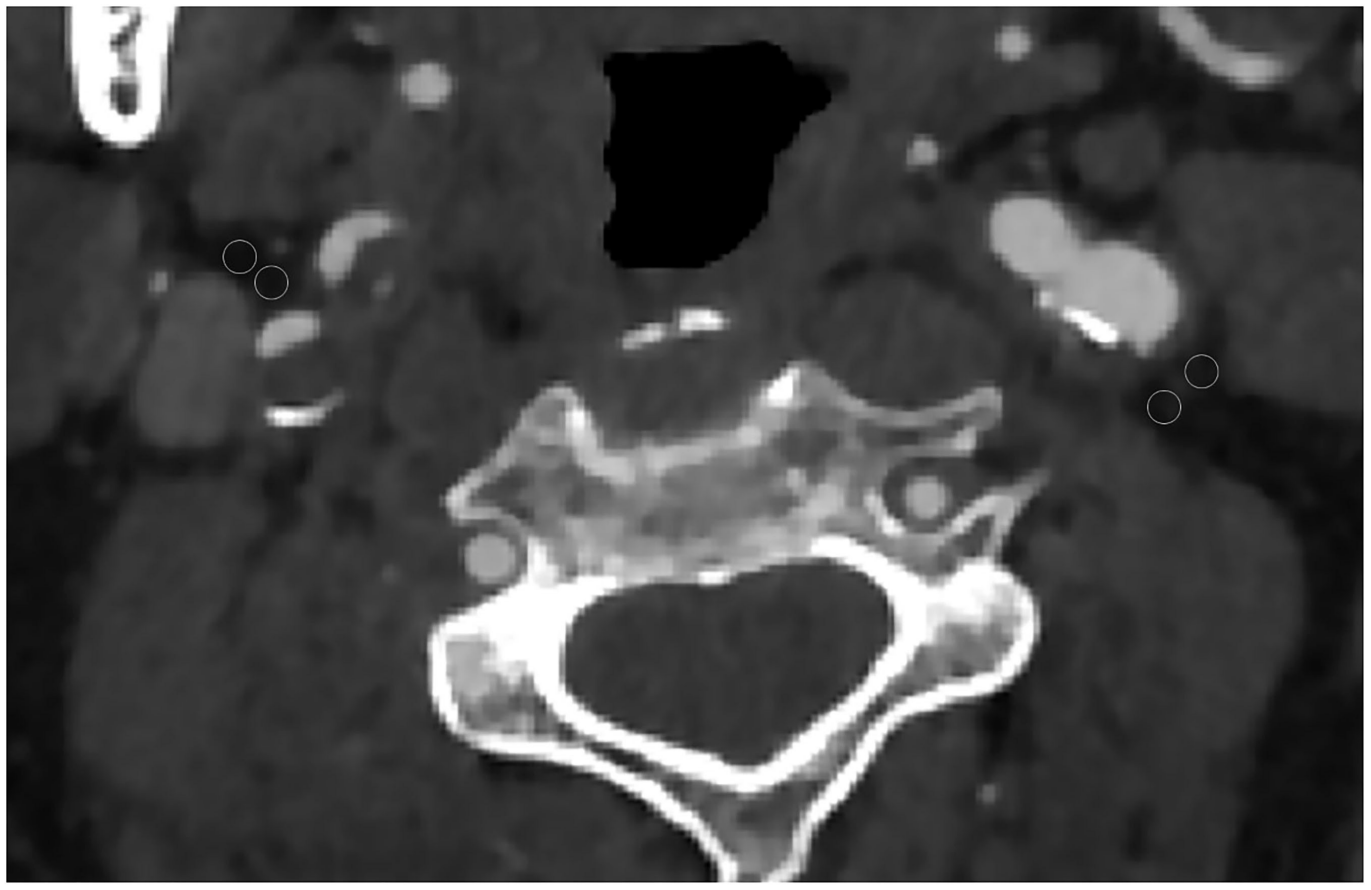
Axial CT angiography image of a 65-year-old male patient. Two regions of interest (ROIs) were placed in the perivascular fat for measured perivascular fat density. In this case, the 2 left ROIs were −60 and −68 and the 2 right ROIs were −62 and −73.
